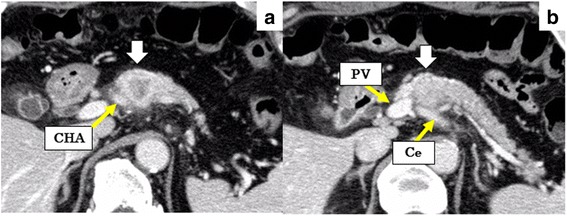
Abdominal CT scan at the initial diagnosis at the previous hospital. The white arrows indicate the pancreatic tumor. a A 26-mm hypovascular tumor in contact with the common hepatic artery (CHA) (> 180°) was seen in the pancreatic body. b The main tumor contacted the PV and bifurcation of Ce. CHA common hepatic artery, Ce celiac artery, SA splenic artery, PV portal vein
